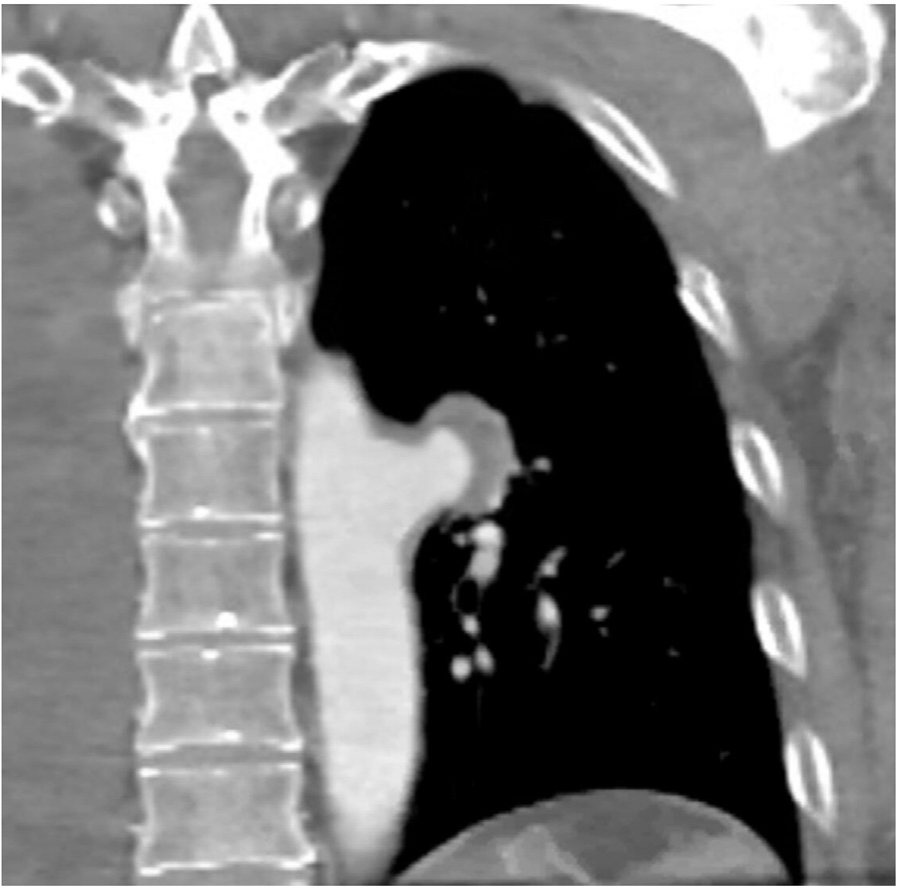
Computed tomography angiography (CTA) displaying coronal view of a saccular, 2.4 × 2.9 × 2.5 cm, mycotic aneurysm of the descending thoracic aorta.
